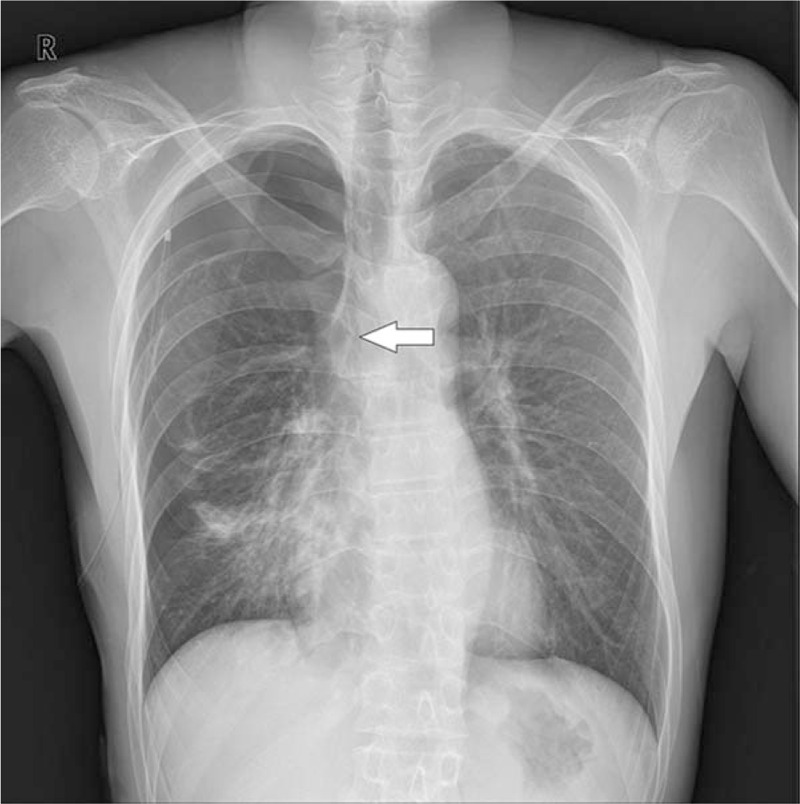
Preoperative chest radiograph shows tracheal bronchus in the right upper lobe with right spontaneous pneumothorax treated with a closed thoracostomy (arrow).
